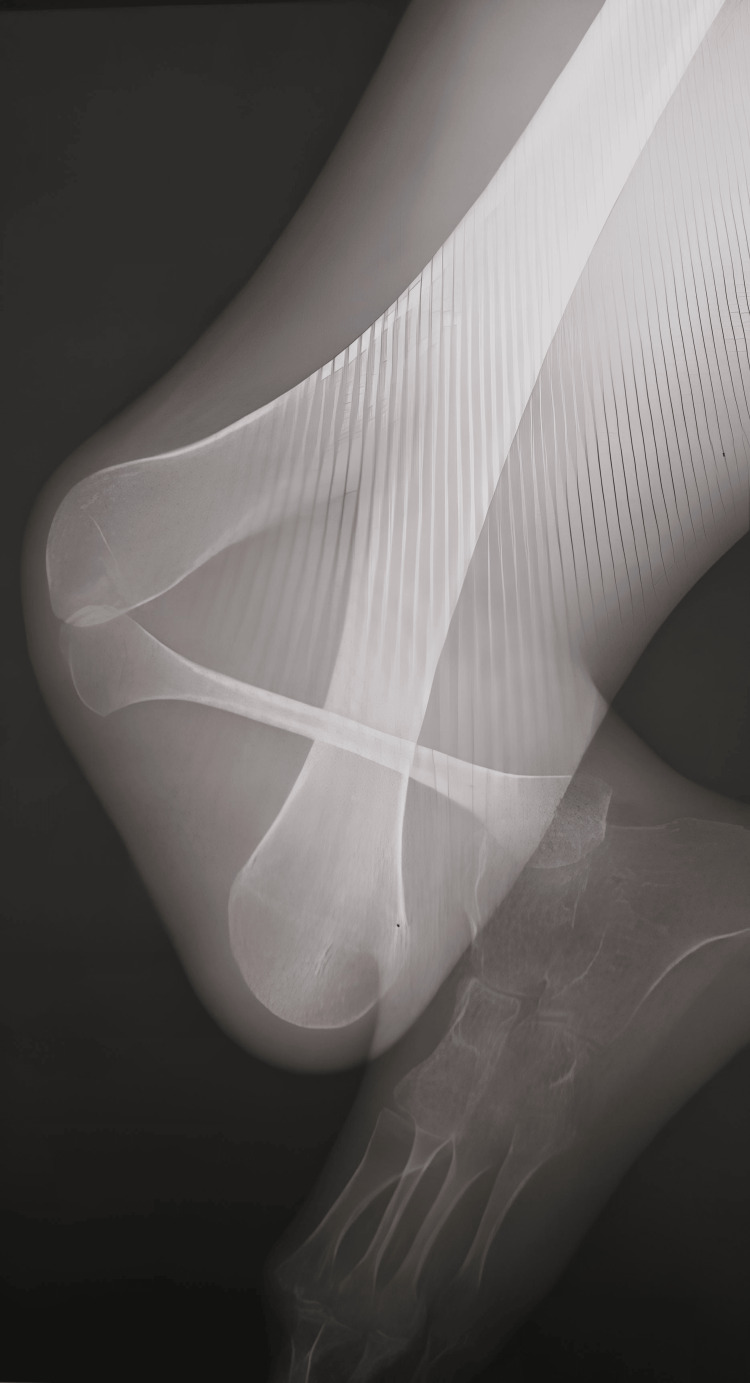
Anteroposterior radiograph of the right lower limb showing a duplicated distal femur (bifurcated femur) and complete tibial aplasia, consistent with GWC GWC: Gollop-Wolfgang complex
